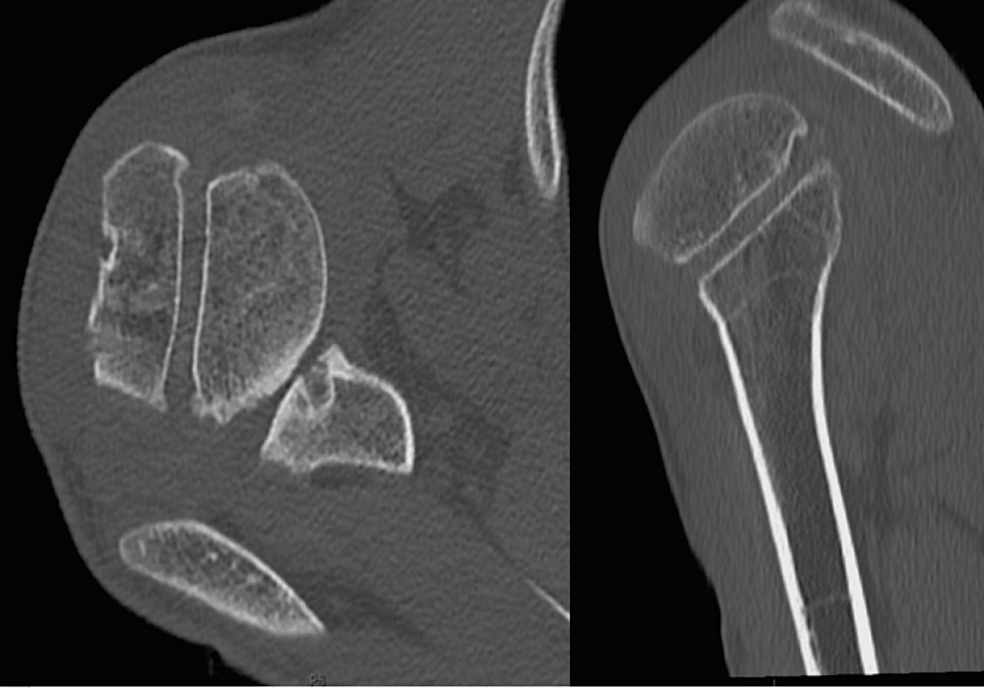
CT scan showing the tunnel drilled in the humeral head to pass the transplant
